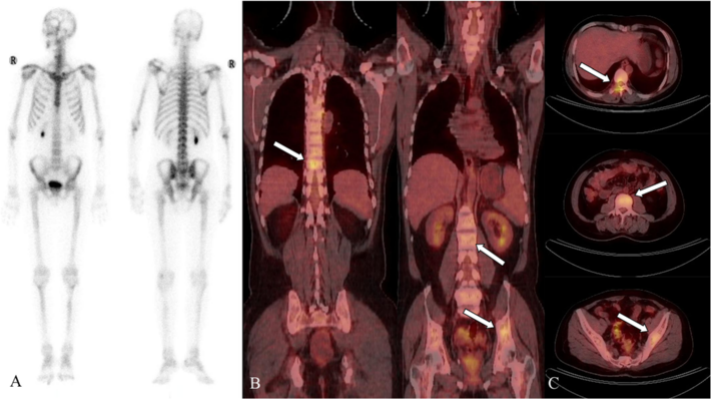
A 32-year-old man with an unknown primary lesion and multiple bone metastases. There was no focally increased uptake detected in the bone scanning (BS) image (A). PET-CT revealed that the bone substance of the ninth thoracic vertebral body and its adjunct, the third lumbar vertebral body and left ilium, was destroyed. ^18^FDG uptake in these areas was significantly increased. The maximum standardized uptake value is 3.96 (B and C). More metastatic lesions were detected using ^18^F-FDG PET-CT than 99mTc-MDP BS.
